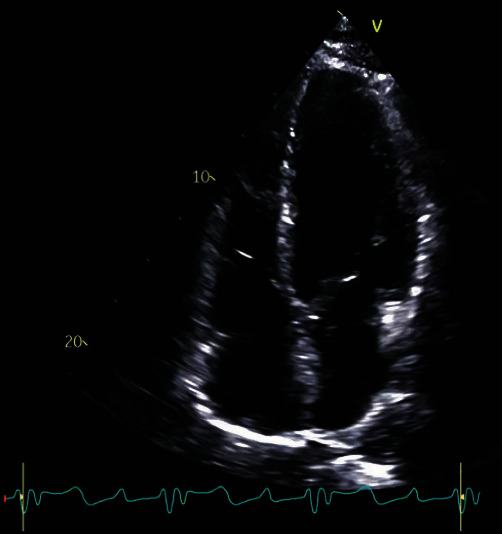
Transthoracic echocardiography showing four chamber cardiac view.
